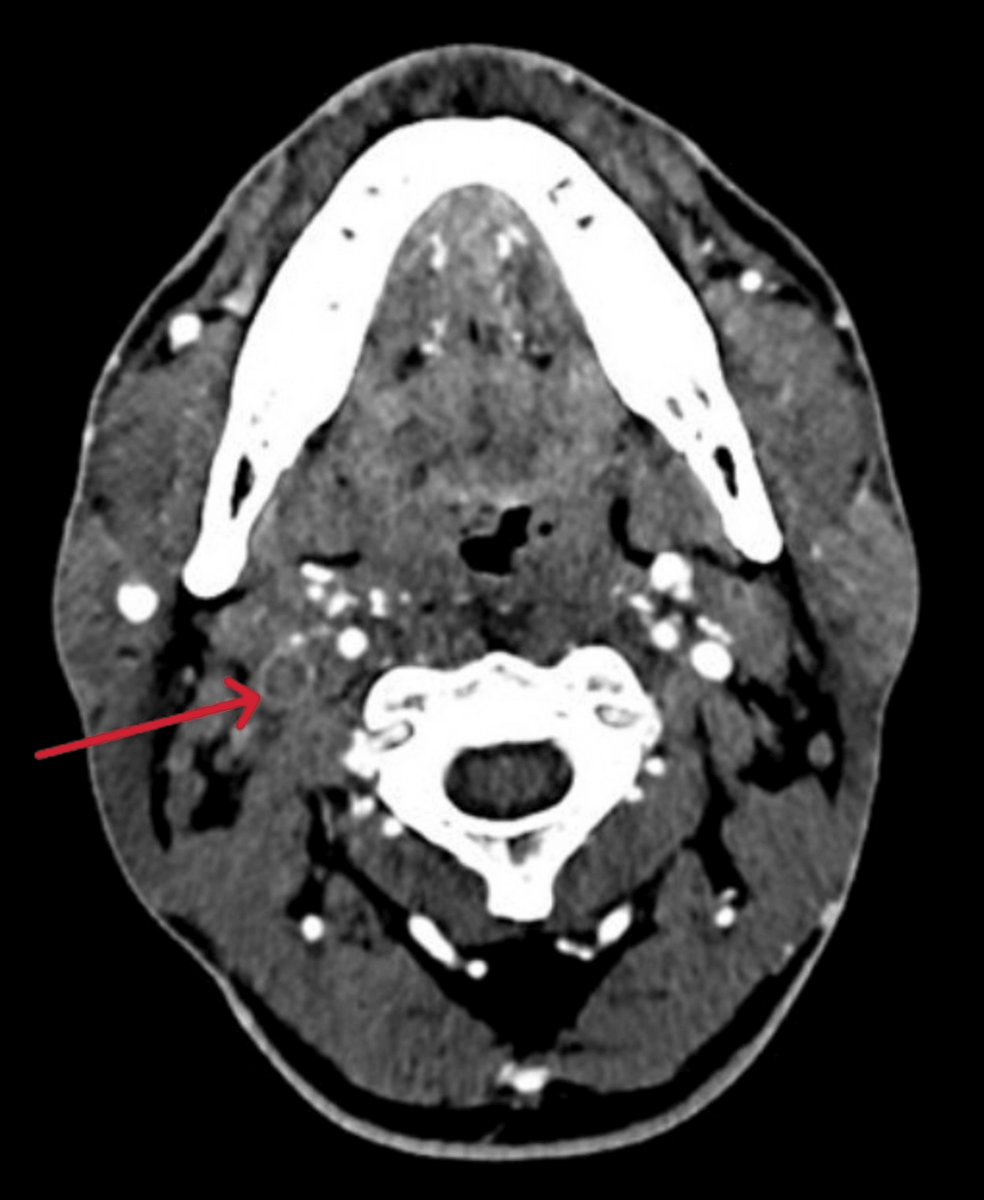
Computed tomography scan (axial view) showing thrombosis of the right internal jugular vein (red arrow)
